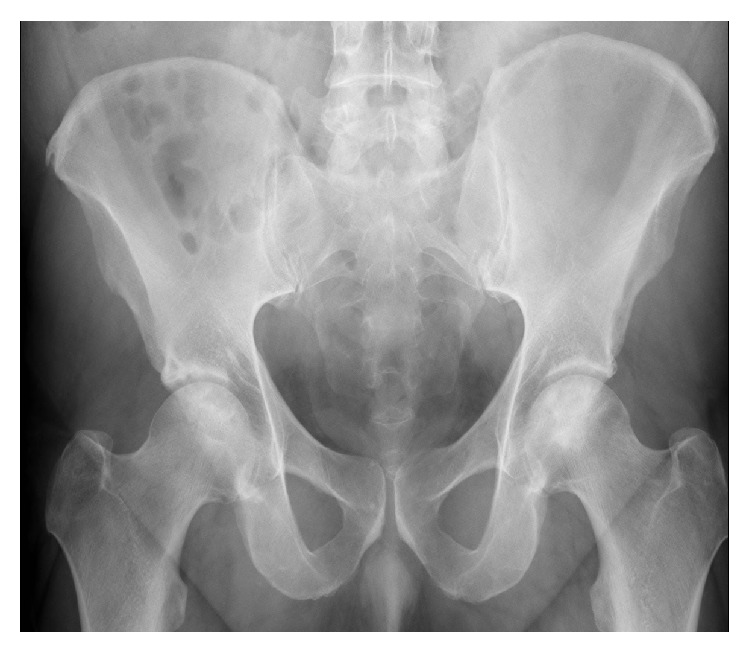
Anterior-posterior hip X-rays in patient number 3 after 9 years on Coumadin are unchanged, Ficat stage II.
